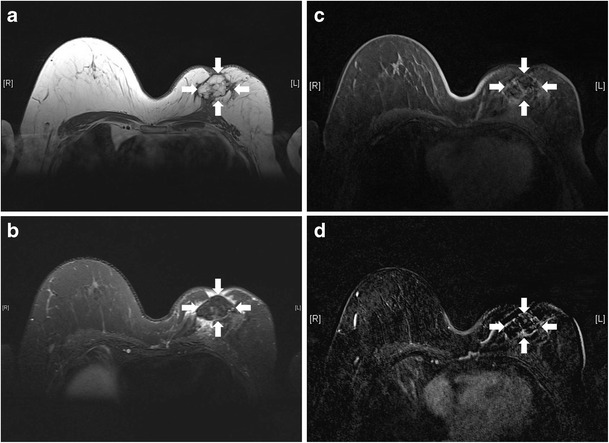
Fat necrosis after autologous fat implant. T1-weighted (a), T2-weighted, fat-saturated (b), and T1-weighted, fat-saturated (c) axial MR images show a multilobulated lesion (arrows) with signal identical to fat in the left breast. Mild perifocal edema is noted (b). T1-weighted, post-contrast axial MR image with subtraction (d) shows the non-enhancing nature of the fatty masses. A thin rim of enhancement surrounding them is likely due to post-operative changes
